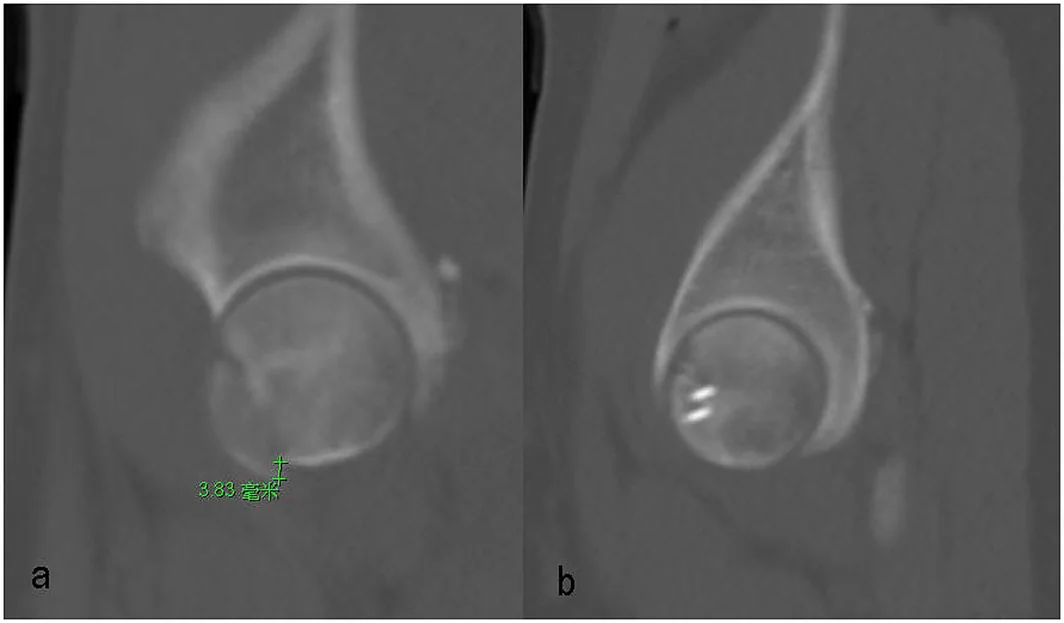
Measurement of maximal residual displacement on CT scans preoperatively (a) and postoperatively (b).
